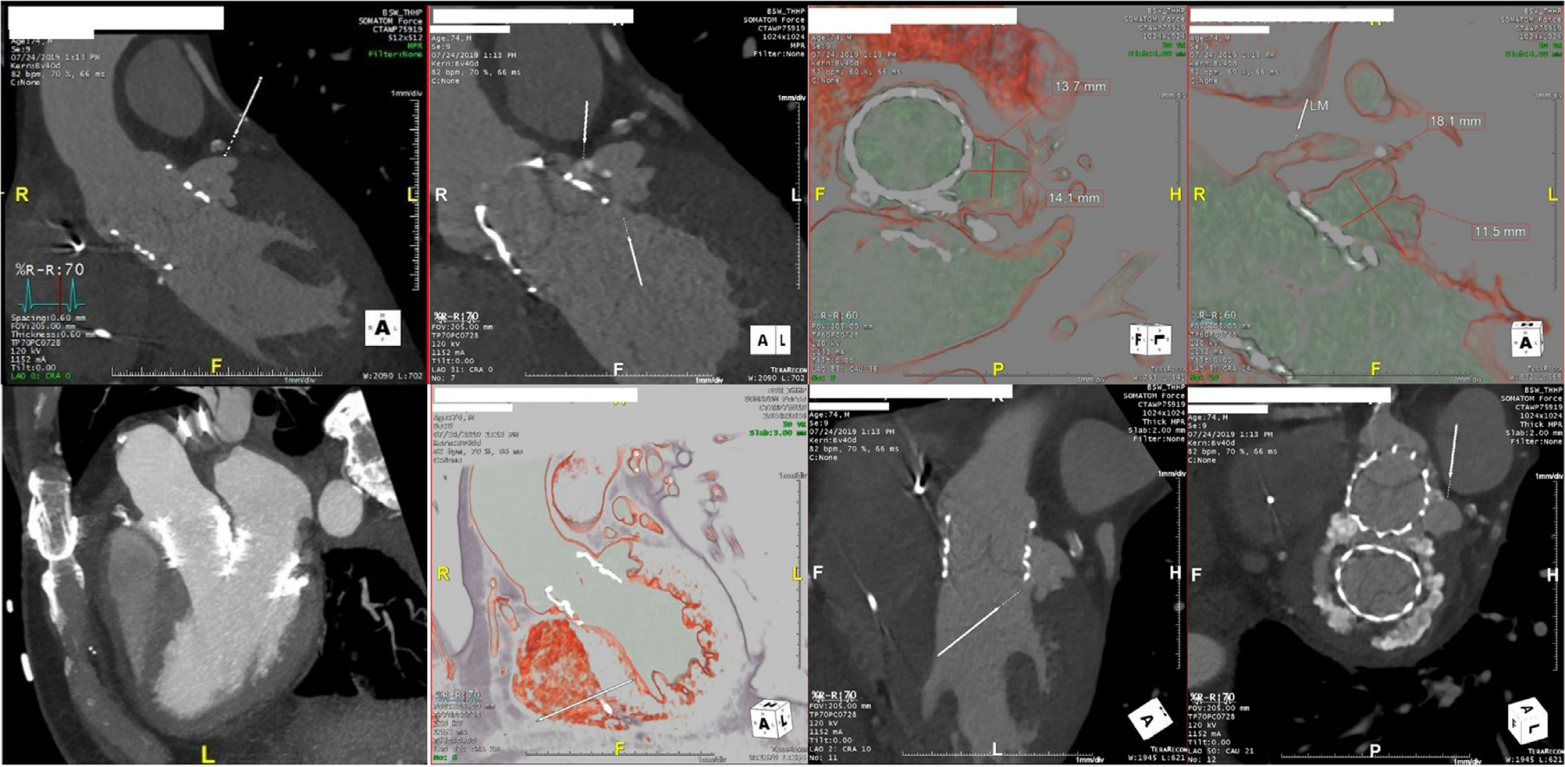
Computed tomography heart after initial surgical repair of the mitral regurgitation and aortic stenosis with Sapien 3 valve in the mitral and aortic position. Aortic annular rupture with significant aorta to left ventricle pseudoaneurysm adjacent to Sapien valve in the aorta (white arrows).
